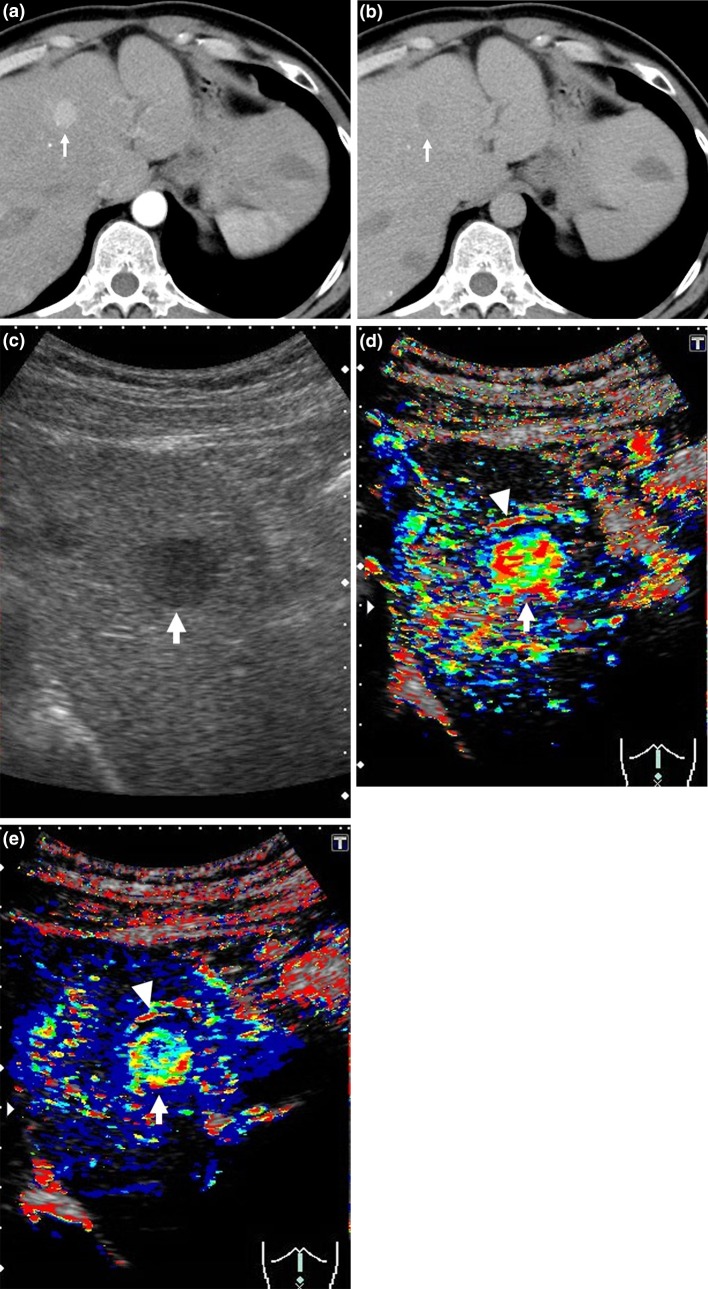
Clinical example of MT (+) group. The patient was a 50-year-old male with chronic hepatitis B virus. Sorafenib administration (400 mg/day) was started for advanced hepatocellular carcinoma (HCC). a Dynamic computed tomography (CT) scan in arterial phase before treatment showed a hypervascular lesion measuring 18 mm in diameter in S4 (*arrow*), which was the target lesion. b Dynamic CT scan in equilibrium phase before treatment showed a hypoattenuating lesion in S4 (*arrow*). c Gray-scale ultrasonography showed a low echoic tumor measuring 18 mm in diameter in S4 (*arrow*). This tumor was established as a target lesion. d The *color* mapping image before treatment showed primarily *red*, *yellow*, or *green* in the tumor (*arrow*). A large artery near this tumor was regarded as the reference point (*arrow head*). e The *color* mapping image 2 weeks after treatment showed primarily *light blue* or *blue* in the tumor (*arrow*). The same artery before treatment was regarded as the reference point (*arrow head*)
